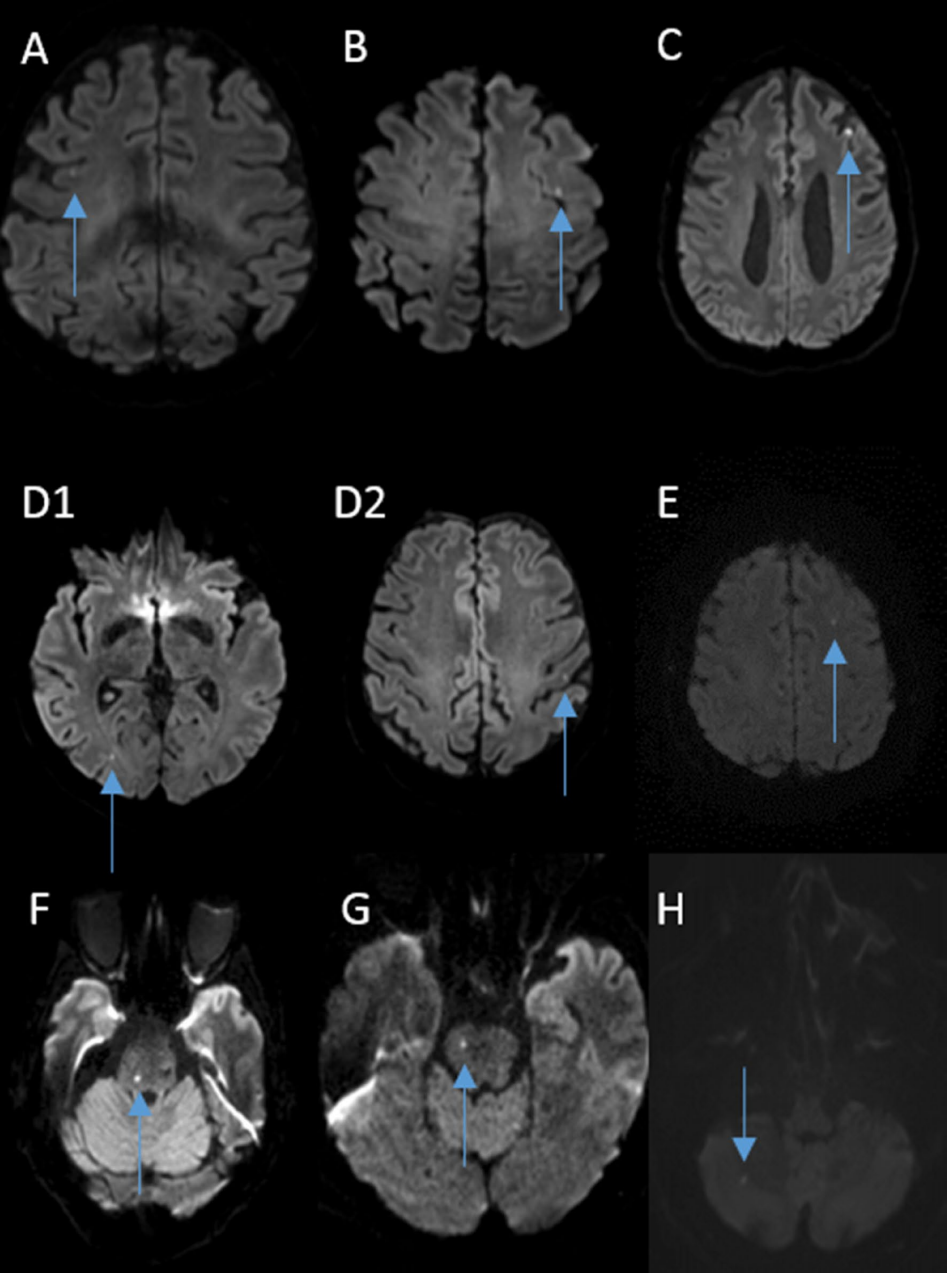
A–H Eight patients with acute ischemic DWI lesions in addition to the hippocampal lesion. A–E Five patients with ischemic lesions in the anterior circulation. D1–D2 Same patient with two different ischemic lesions. F–H Three patients with additional lesions in the posterior circulation
